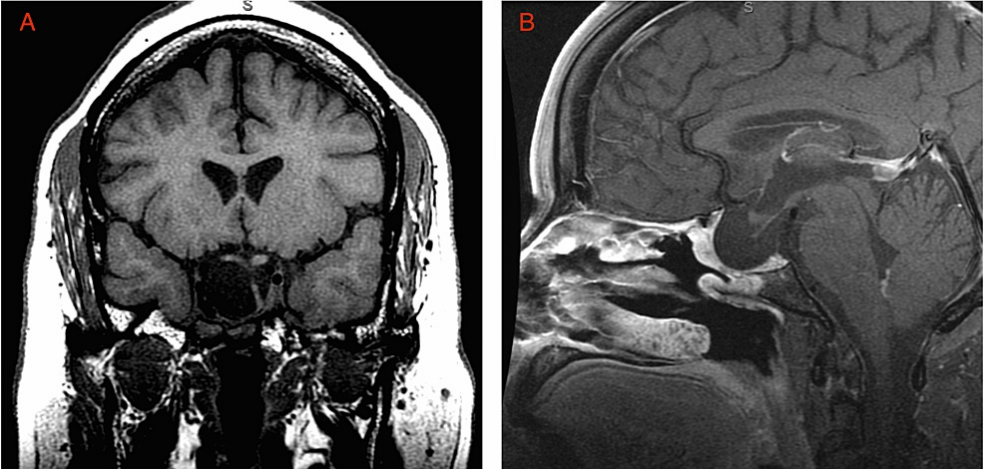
MRI of the brain MRI of the brain in the coronal section (A) and sagittal section (B) showed complete resection of the pituitary macroadenoma with postoperative changes.
